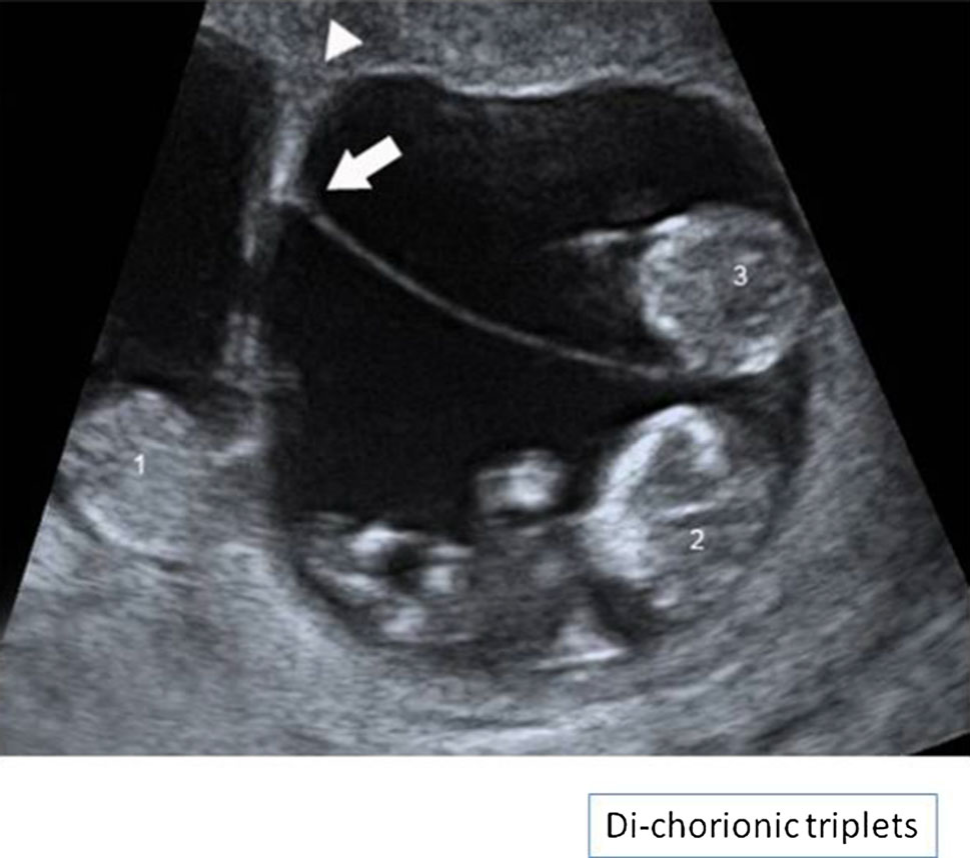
Ultrasound picture of a dichorionic, triamniotic trio at 13 weeks gestational age. The arrow indicates the amniotic membranes of fetuses 2 and 3, which are a monozygotic pair. At this time, it is unsure if Fetus 1 shares zygosity with fetuses 2 and 3. Numbers indicate the three fetuses. (Used with permission from Lamb et al. 2012)
